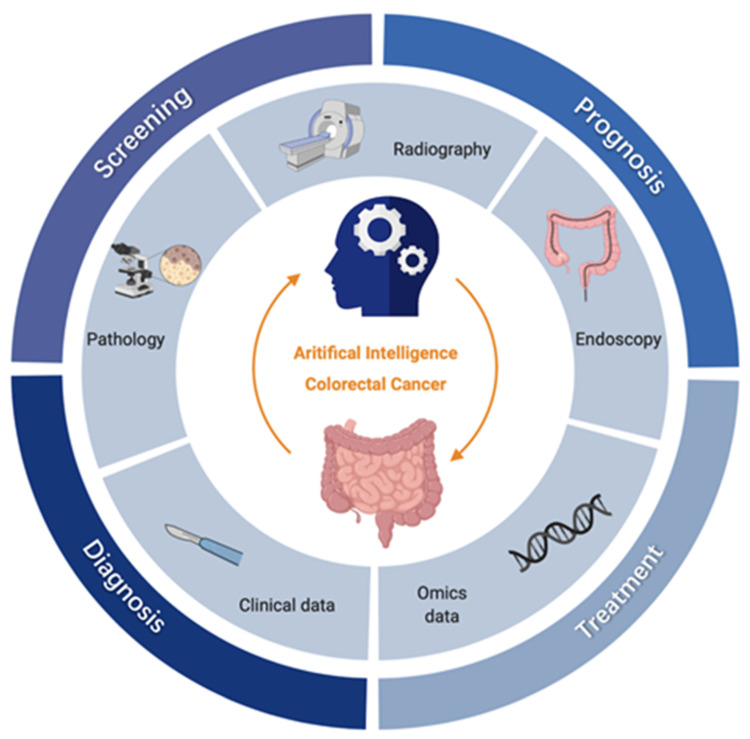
Clinical applications of AI for CRC. The inner circle represents the main data types in CRC research, including radiological images (i.e., Computed Tomography (CT), Magnetic Resonance Imaging (MRI), etc), endoscopic images, pathological images, clinical data, and omics data; the outer circle represents the four key clinical parts of CRC, i.e., screening, diagnosis, treatment, and prognosis; for each clinical part, AI has subdivided and specific tasks, which are shown in boxes outside the circle respectively. OS, overall survival; DFS, disease-free survival; nCRT, neoadjuvant radiotherapy.
